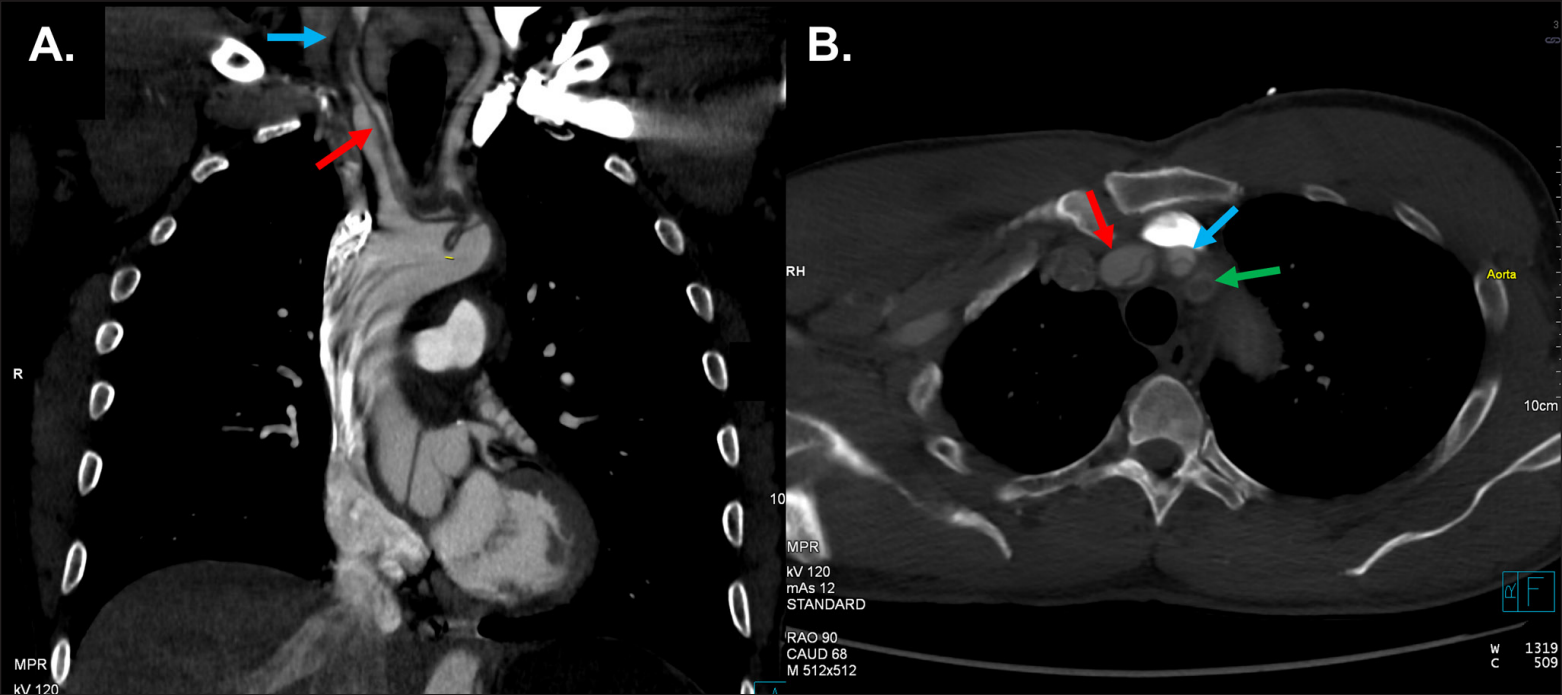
Supra-aortic vessel dissection. Sagittal (A) and transverse (B) computed tomography showing brachiocephalic artery dissection (red arrow) and near complete occlusion of the right common carotid artery (blue arrow) due to thrombosis of the false lumen. Dissection of the right subclavian artery (green arrow) is also seen.
